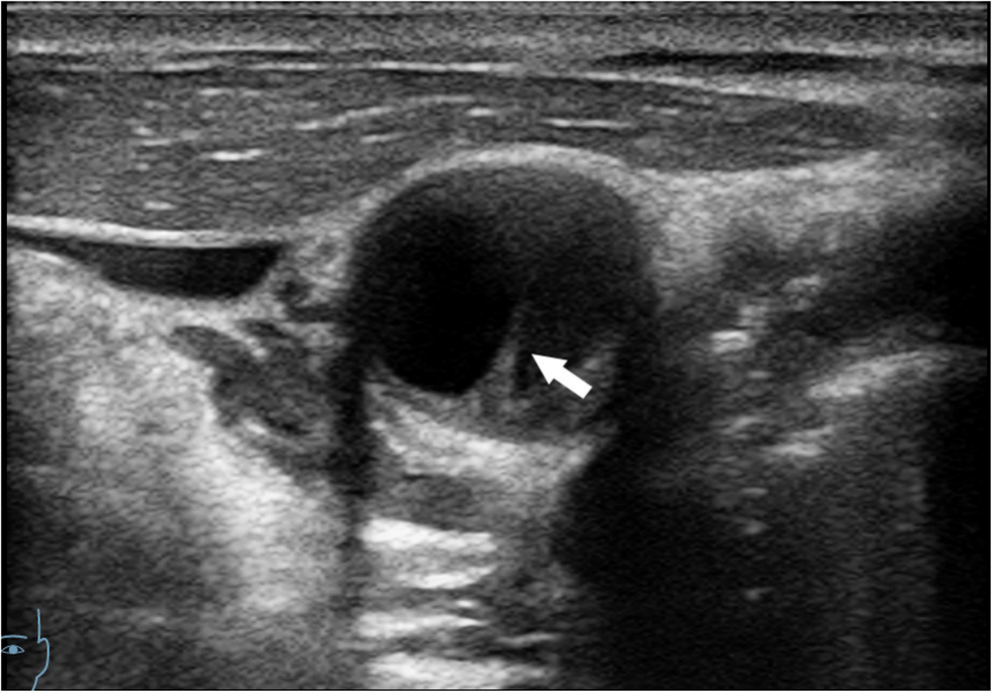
Conventional ultrasound showing axial view of CaW (arrow indicates shelf-like CaW).
